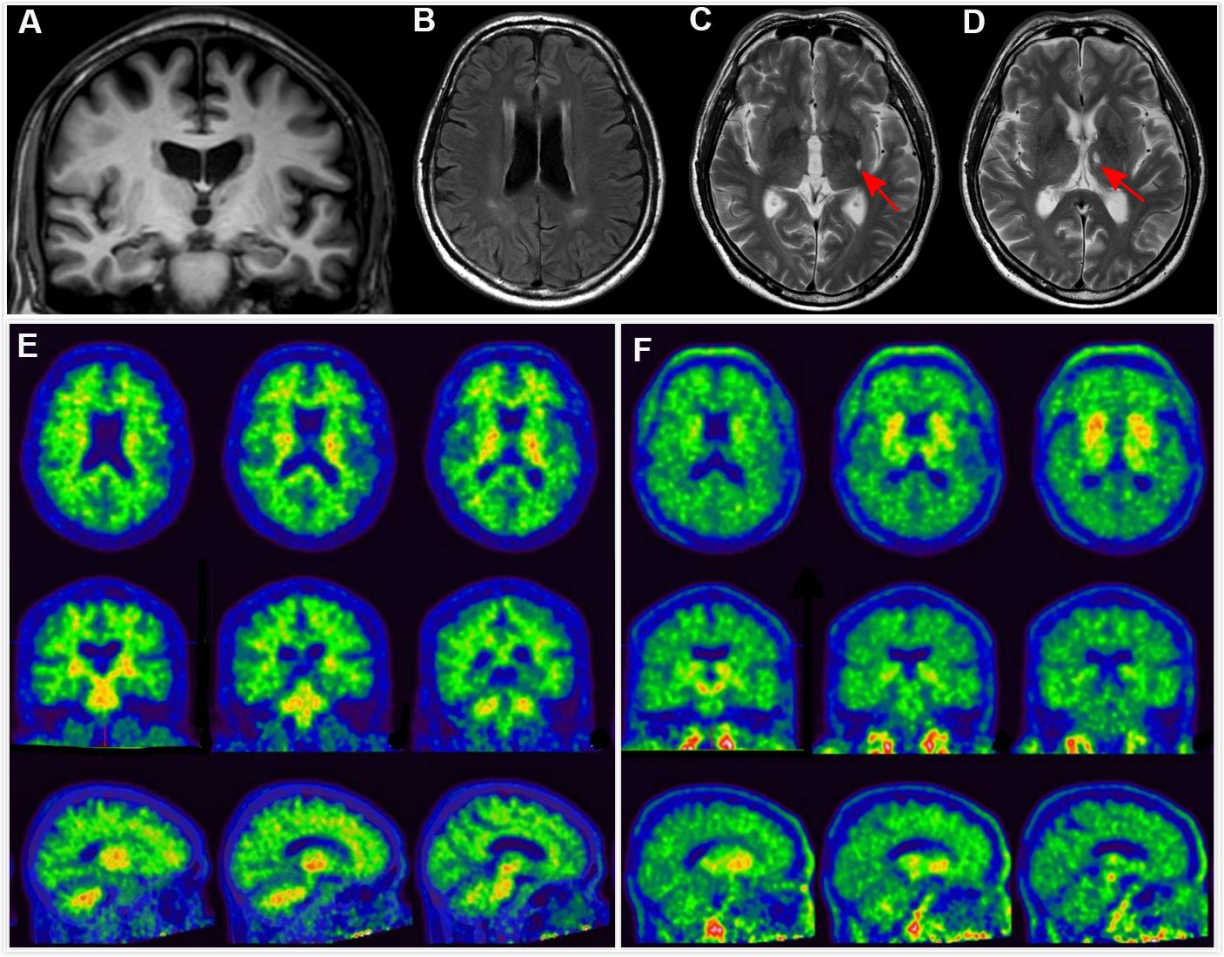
Clinical utility of AD-RAI in MCI subjects. A 68-year-old man with 11 years of education had complaints of memory decline for over 3 years. Z-score in Trial 4 of HKLLT was -1.94 SD (≤ -1 SD, i.e. MCI). Visual MTA rating score on MRI was 1 (≥ 1), (A) which was suggestive of AD. However, HV measures yielded conflicting results, with HF of 0.47% (> 0.41%) and raw HV of 7.38ml (> 6.07mL) suggestive of non-AD. FLAIR and T2-weighted sequences showed periventricular white matter hyperintensity and two subcortical lacunes (red arrows) (B–D). AD-RAI was only 0.11 (< 0.5) also suggestive of non-AD. Subsequent PIB PET (E) and T807 PET (F) showed negative results (i.e. A-T-), supporting the finding of AD-RAI. The MCI syndrome and mild MTA might be associated with cerebral SVD (i.e. vascular MCI associated with SVD).
